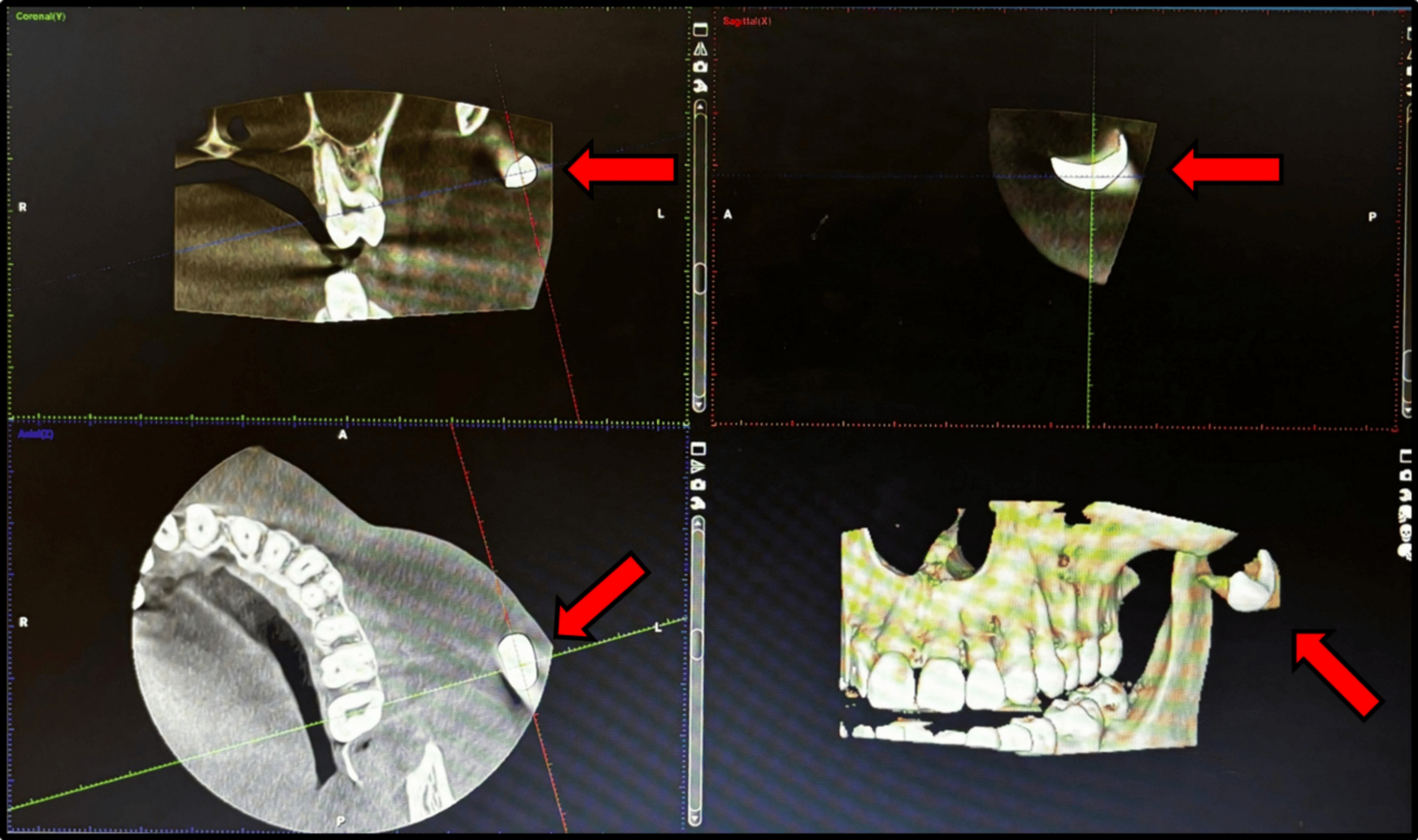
Cone beam computed tomography (CBCT) showing foreign body over left malar region CBCT image in different sections
